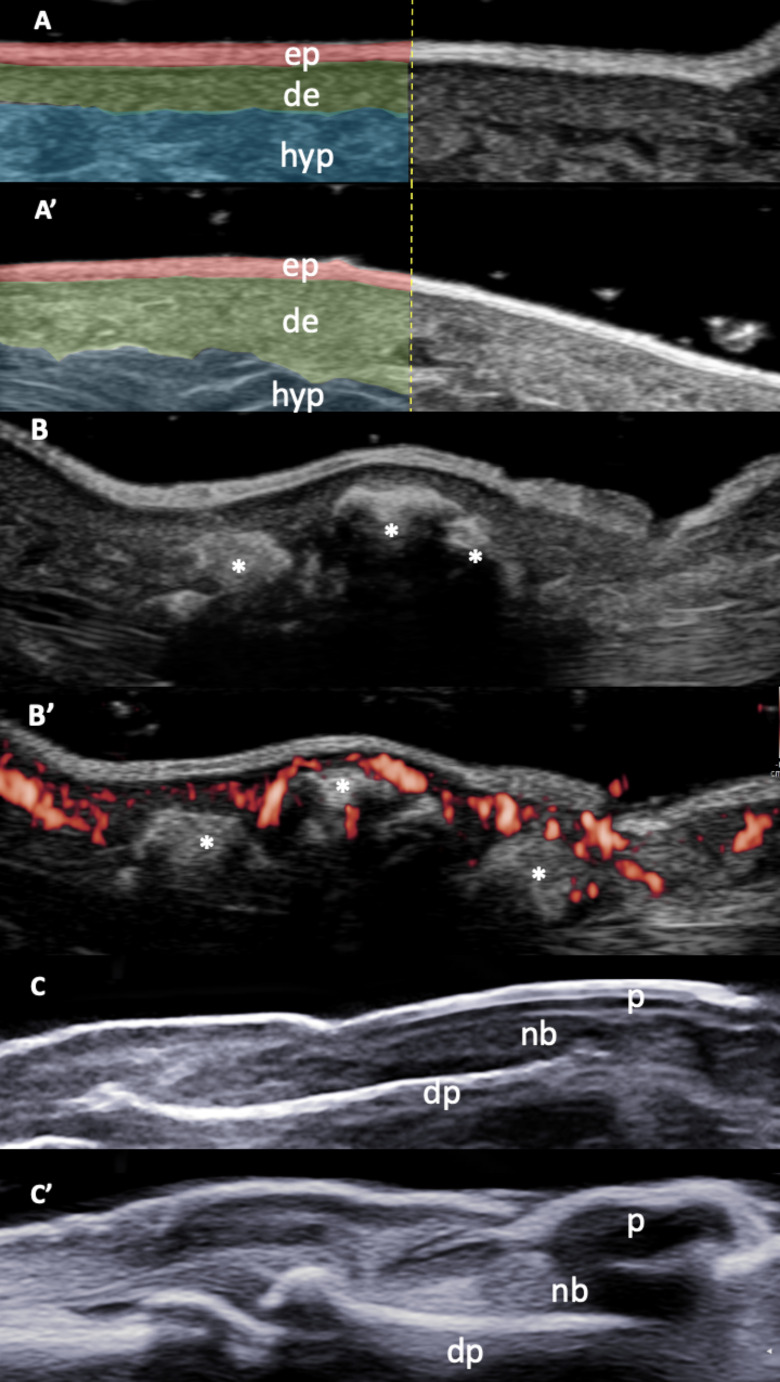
Ultrasound in the assessment of skin (scleroderma), sub-cutaneous soft tissues (calcinosis cutis) and nails (nail psoriasis). A presents a comparative ultrasound image of skin layers in the atrophic phase of systemic sclerosis, obtained with a high-frequency probe (22 MHz). The epidermis (red) appears as a thin hyperechoic layer, while the dermis (green) is hypoechoic with tightly packed, regular connective tissue fibers. The subcutaneous tissue (blue) is more hypoechoic, with looser connective tissue fibers. In A’, the epidermal, dermal, and subcutaneous layers of a healthy subject are clearly delineated, following the same echogenic patterns as in A, though with notable differences in thickness, especially in the dermis. B shows an ultrasound image of subcutaneous calcinosis, highlighting calcifications of variable shapes and sizes (asterisks) accompanied by acoustic shadowing. In B’, a surrounding power Doppler signal (red spots) is visible in a patient with systemic sclerosis. Finally, C provides an ultrasound comparison of a physiological nail, showing a normal trilaminar structure, while C’ depicts a psoriatic nail with loss of the ventral plate and increased nail bed thickness. *de* = dermis; *dp* = distal phalanx; *ep* = epidermis; *hyp* = hypodermis; *nb* = nail bed; *p* = nail plate. A, A’, B, and B’ kind courtesy of Prof. L. Idolazzi
